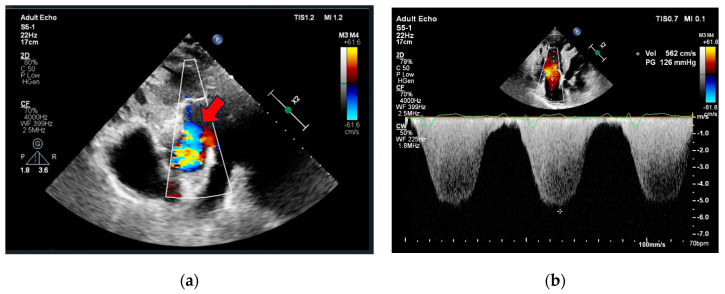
Echocardiography (a) parasternal short axis view showing aortopulmonic window (red arrow), (b) apical 4-chamber view showing right ventricle dilatation, hypertrophy, severe tricuspid regurgitation, and pulmonary hypertension.
